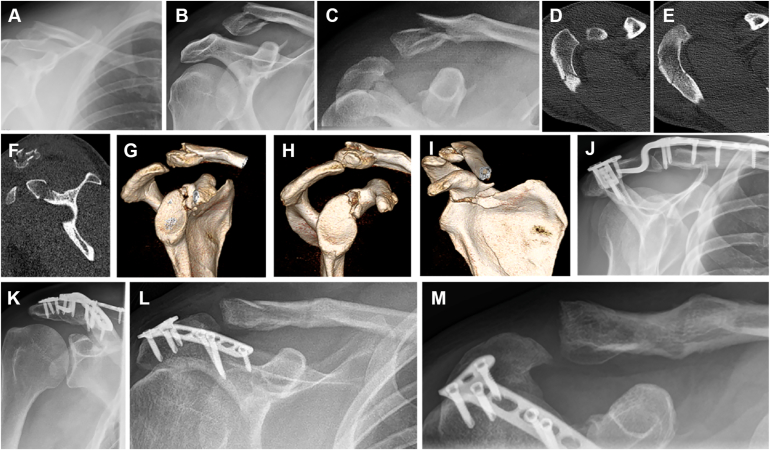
Triple disruption injury of SSSC and its treatment. The injury occurred in a 38-year-old patient following a high-energy bicycle accident. (A–I) Initial radiographic, 2- and 3-dimensional CT images demonstrating a triple disruption of the SSSC of the right shoulder, including fractures of the lateral clavicle, acromion, and the base of the coracoid process. (J and K) Post-operative radiographs obtained 2 months after open reduction and internal fixation, prior to implant removal. Fixation included a 3.5-mm T-shaped locking compression plate (LCP-plate) for the acromion and a hook plate for the lateral clavicle fracture. (L and M) Radiographs at 6-month follow-up showing complete fracture union, proper alignment, and absence of implant-related complications following removal of the hook plate 4 months before. *SSSC*, superior shoulder suspensory complex; *CT*, computed tomography. A triple disruption of the right SSSC involving fractures of the lateral clavicle, acromion, and base of the coracoid process occurred in a 38-year-old patient after a high-energy bicycle accident. Open reduction and internal fixation were performed using a 3.5-mm T-shaped locking compression plate for the acromion and a hook plate for the lateral clavicle. This strategy restored the dominant unstable components of the SSSC ring and achieved complete fracture union, proper alignment, and no implant-related complications at 6-month follow-up after hook plate removal. The case also reinforces the importance of CT-based pre-operative planning, because the true number and configuration of disruptions may be underestimated on plain radiographs. Overall, the treatment principle for triple disruptions is selective but decisive stabilization. Displaced acromion, clavicle, scapular spine, glenoid neck, and coracoid fractures should be addressed surgically when they contribute to instability, malalignment, impingement, loss of the deltoid lever arm, or inability to begin rehabilitation. Conversely, a minimally displaced or anatomically reduced component may be managed nonoperatively if fixation of the dominant unstable structures restores ring stability and intraoperative or radiographic assessment confirms acceptable alignment. Thus, the decision should not be based solely on the number of disrupted structures, but on which components are mechanically responsible for persistent instability. The treatment principle for quadruple disruptions is individualized restoration of functionally relevant stability. In young, active, high-demand, or reconstructable patients, early operative fixation of the dominant unstable components should be strongly considered to restore SSSC continuity, glenohumeral congruity, scapulothoracic mechanics, and the ability to begin rehabilitation. In elderly, low-demand, medically complex, or osteoporotic patients, nonoperative treatment may be reasonable when alignment is acceptable, pain is manageable, neurovascular status is intact, and the expected morbidity of extensive surgery outweighs the likely functional benefit. Therefore, the central decision is not simply whether 4 structures are disrupted, but whether the remaining shoulder girdle can maintain alignment, permit functional recovery, and avoid chronic instability without operative reconstruction. *CT*, computed tomography; *SSSC*, superior shoulder suspensory complex.
